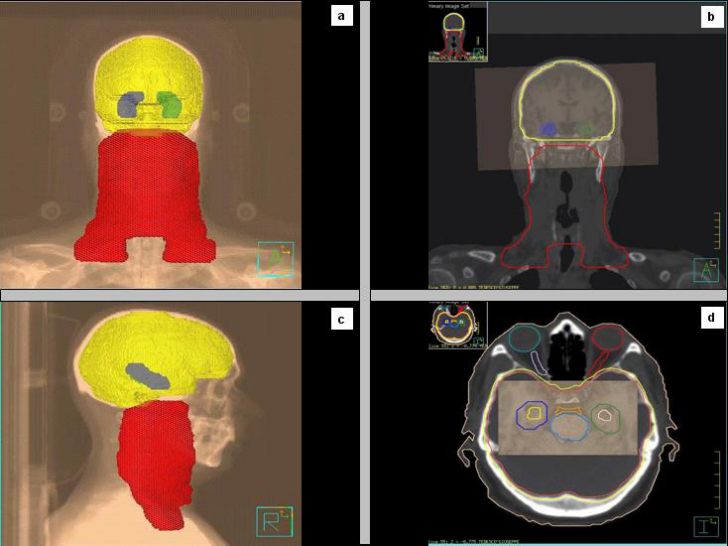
Target volumes including the head and neck region and the whole brain with concomitant sparing of the bilateral hippocampal regions (Figure 3a-c); fusion MRI employed for appropriate delineation of the hippocampus (Figure 3b-d).
